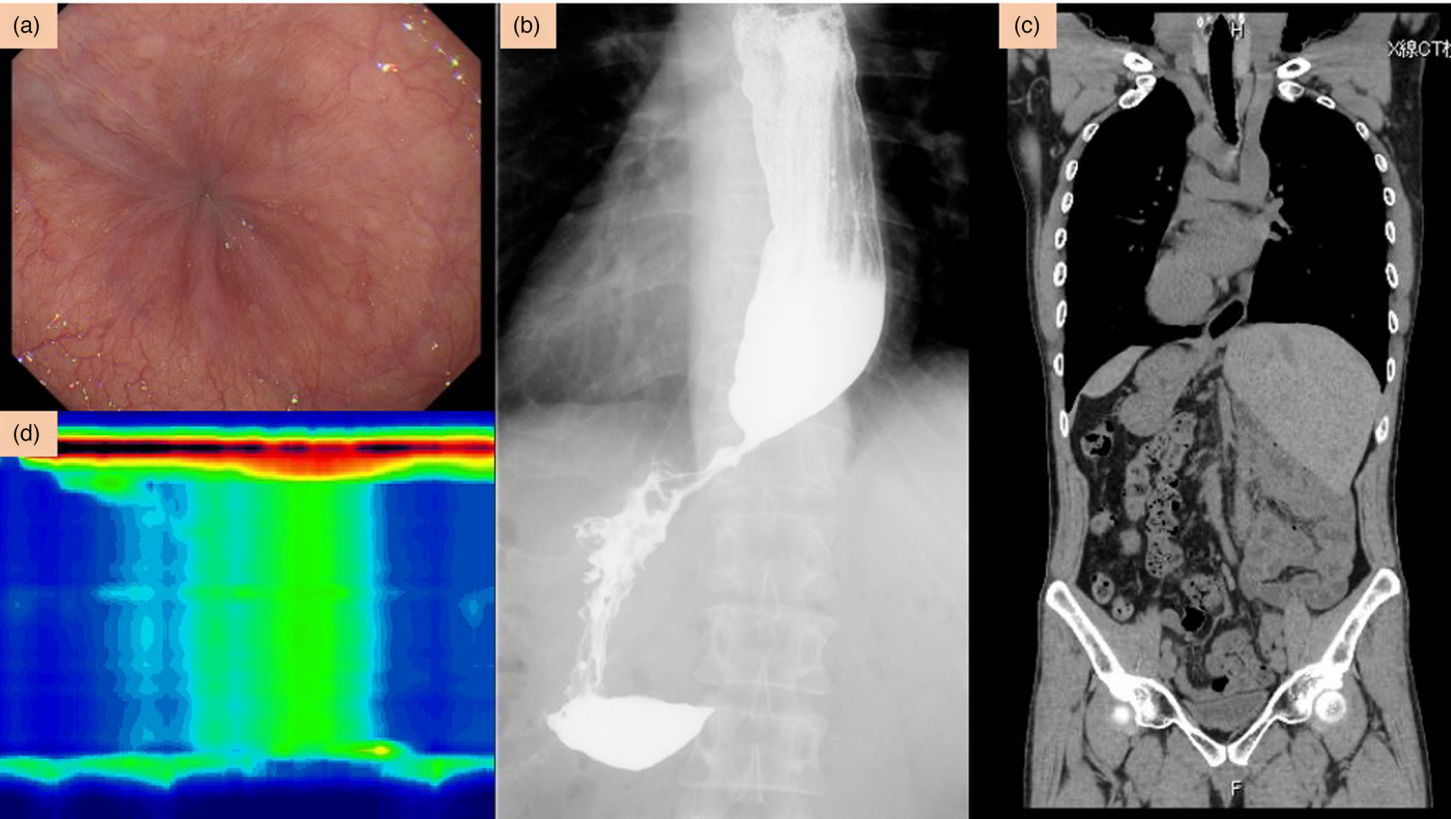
Preoperative evaluation: (a) Endoscopic finding showing a resistance in passing through the gastroesophageal junction (GEJ) as well as the rosette sign. (b) Barium esophagogram reveals a delay in the passage of the contrast and a narrowing at the lower esophageal sphincter (LES), creating the classic “bird's beak sign.” Noticeably, the barium esophagogram findings seemed to show an inverted (mirror‐image) positioning of the visceral organs. (c) CT scan shows the completely reversed position of the thoracic and abdominal visceral organs, establishing the diagnosis of situs inversus totalis. (d) High‐resolution manometry findings are consistent with Type II achalasia, showing panpressurization.
